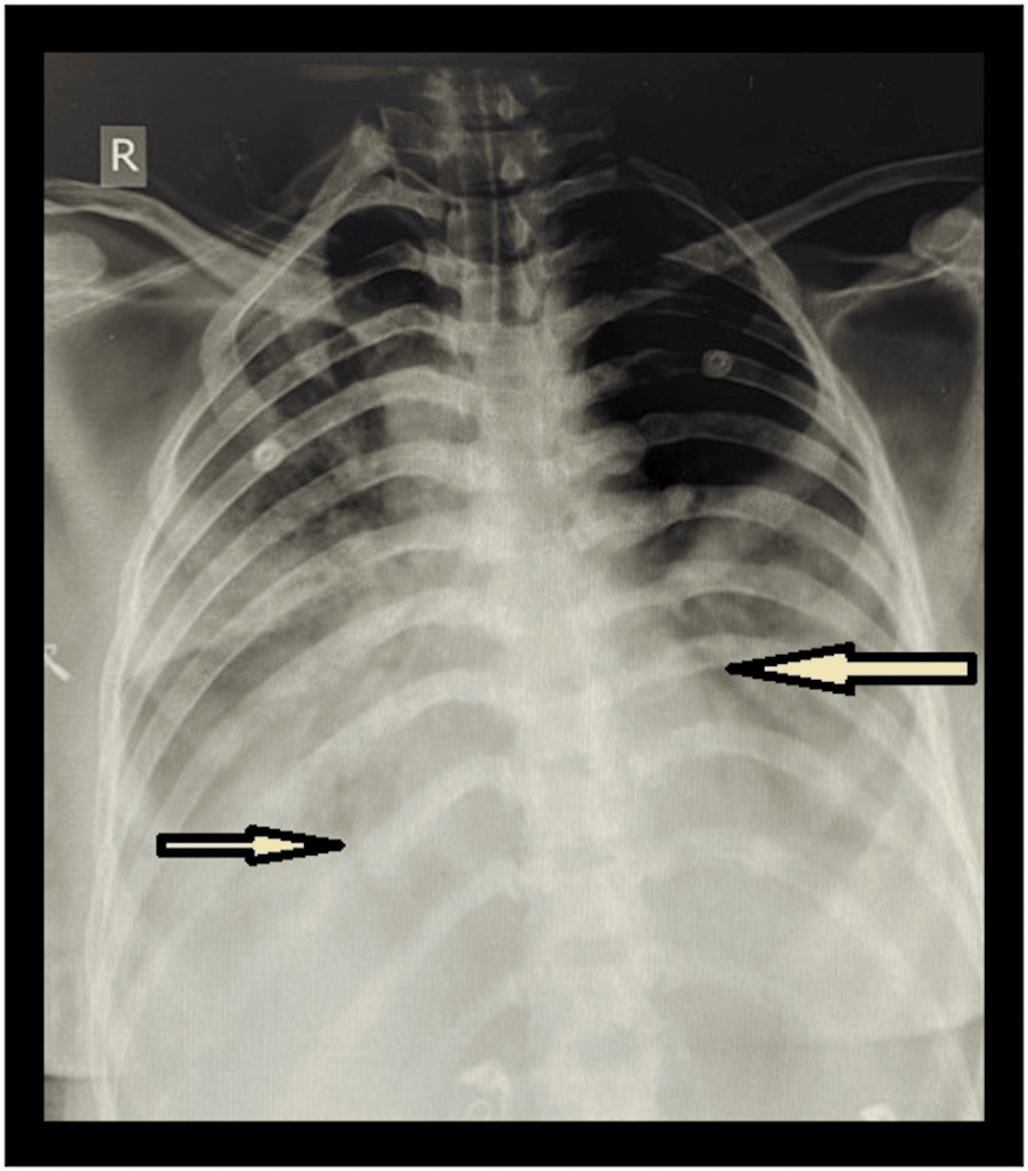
The chest X-ray posteroanterior (PA) view shows non-homogenous opacities with air bronchograms in the bilateral lower zones, suggestive of bilateral lower lobe pneumonia (arrows). The image is original and produced by the authors
